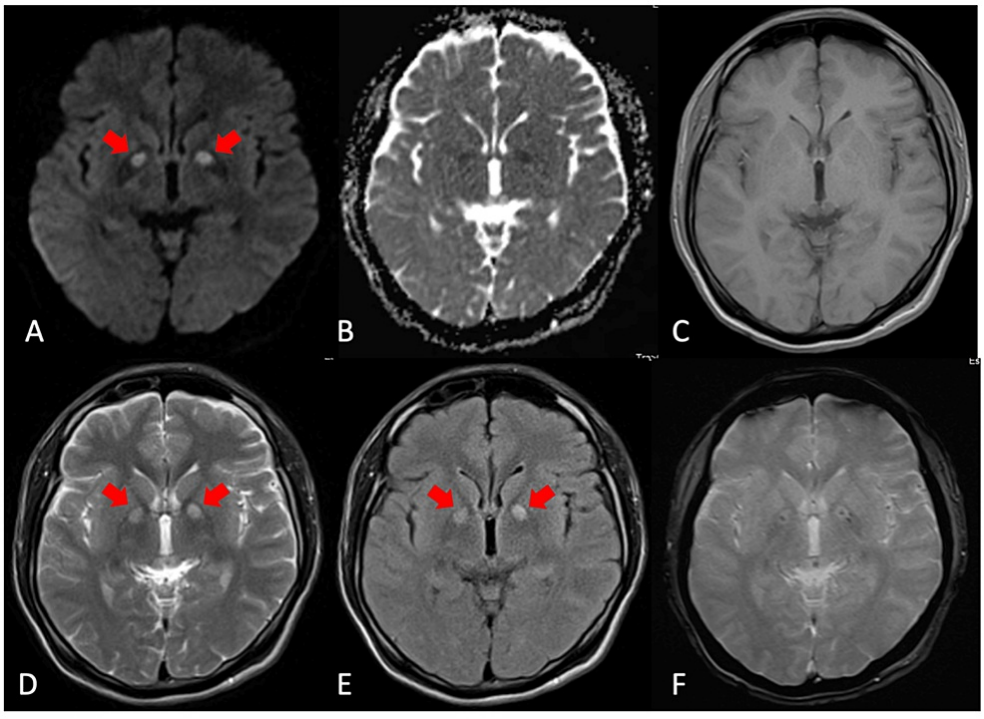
MRI axial, cross-sectional view A) Diffusion-weighted imaging (DWI), B) apparent diffusion coefficient (ADC) map, C) T1-weighted, D) T2-weighted, E) T2 fluid-attenuated inversion recovery (FLAIR), and F) gradient echo (GRE) The red arrows pointing to lesions situated around the bilateral globus pallidus MRI: magnetic resonance imaging
